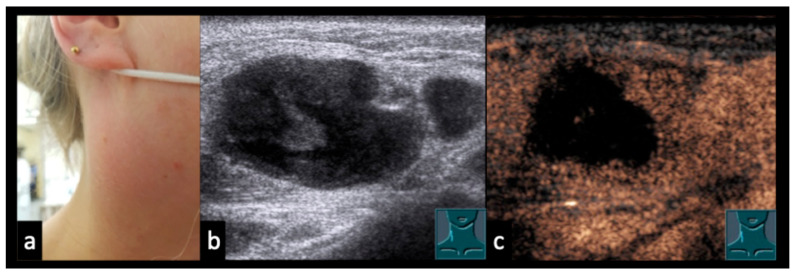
32-year-old patient with a swollen and reddened throat (a), undergoing immunosuppressive therapy for Crohn’s disease and presenting with abscessing lymphadenopathy without detectable bacterial pathogens. (b): B-mode ultrasound shows an inhomogeneous lymph node. (c): CEUS demonstrates partial absence of enhancement, consistent with abscess formation.
